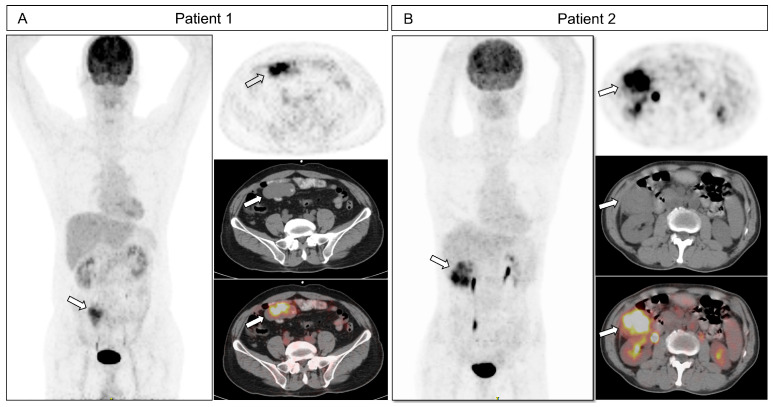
Representative cases. (A) A 67-year-old male diagnosed with a small intestinal GIST (size 6.4 cm, high-risk NIH criteria, white arrow). The SUVmax was 8.5, MTV was 43.52 cm^3^, and TLG was 177.13. The patient overall survival was noted at 67 months after surgery without recurrence. (B) A 69-year-old man with small intestinal GIST (size 6.3 cm, high-risk NIH criteria, white arrow). The SUVmax was 11.37, MTV was 130.0 cm^3^, and TLG was 601.9. The patient died and the overall survival was noted at 24 months after surgery.
